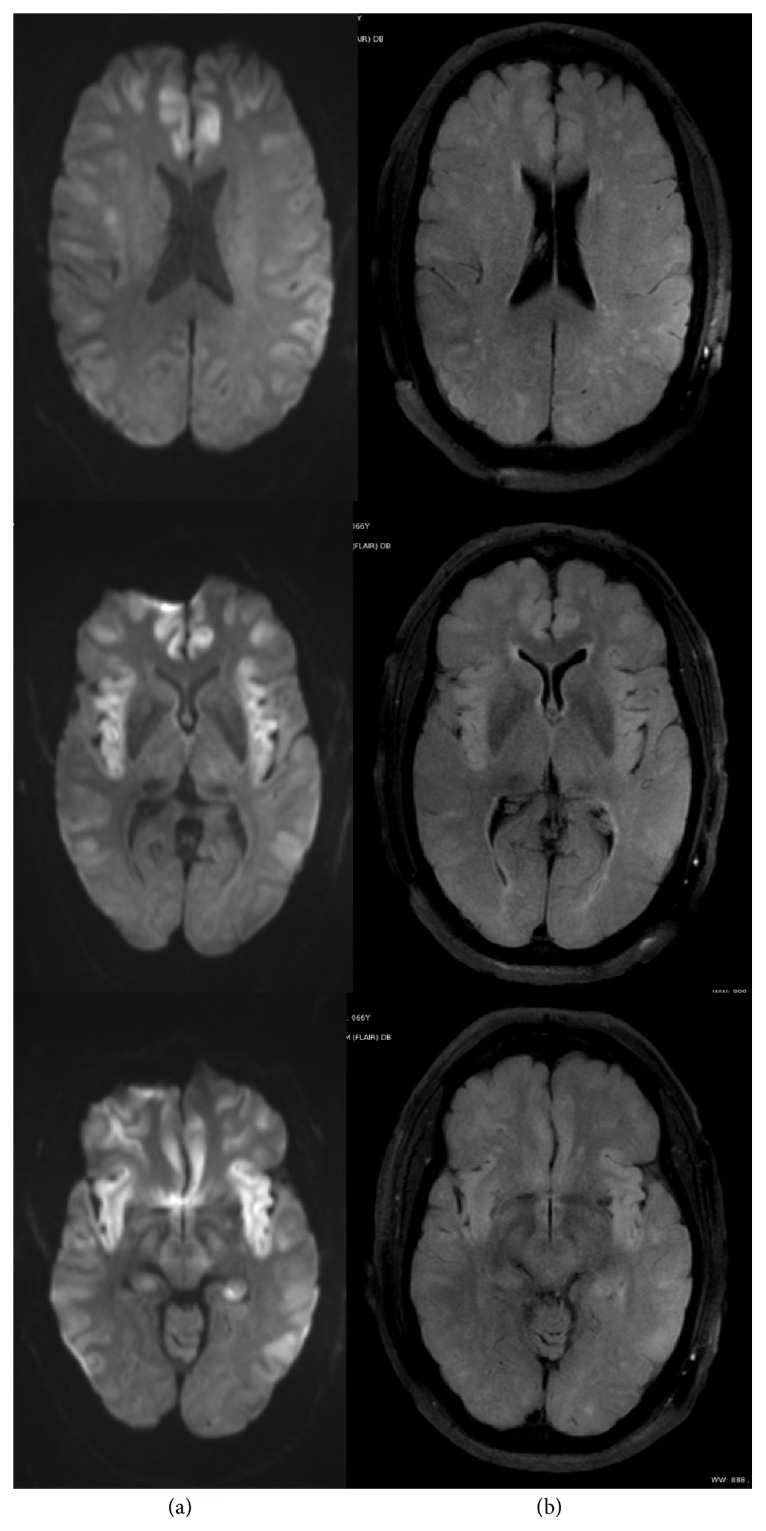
MRI of the brain ((a) DWI sequence, (b) FLAIR sequence) on day 3 after presentation, showing extensive areas of restricted diffusion with associated FLAIR hyperintensity involving bilateral temporal lobes, bilateral insular, bilateral frontal, and parietal regions in cortical and subcortical areas and diffuse mild effacement of the cerebral sulci.
